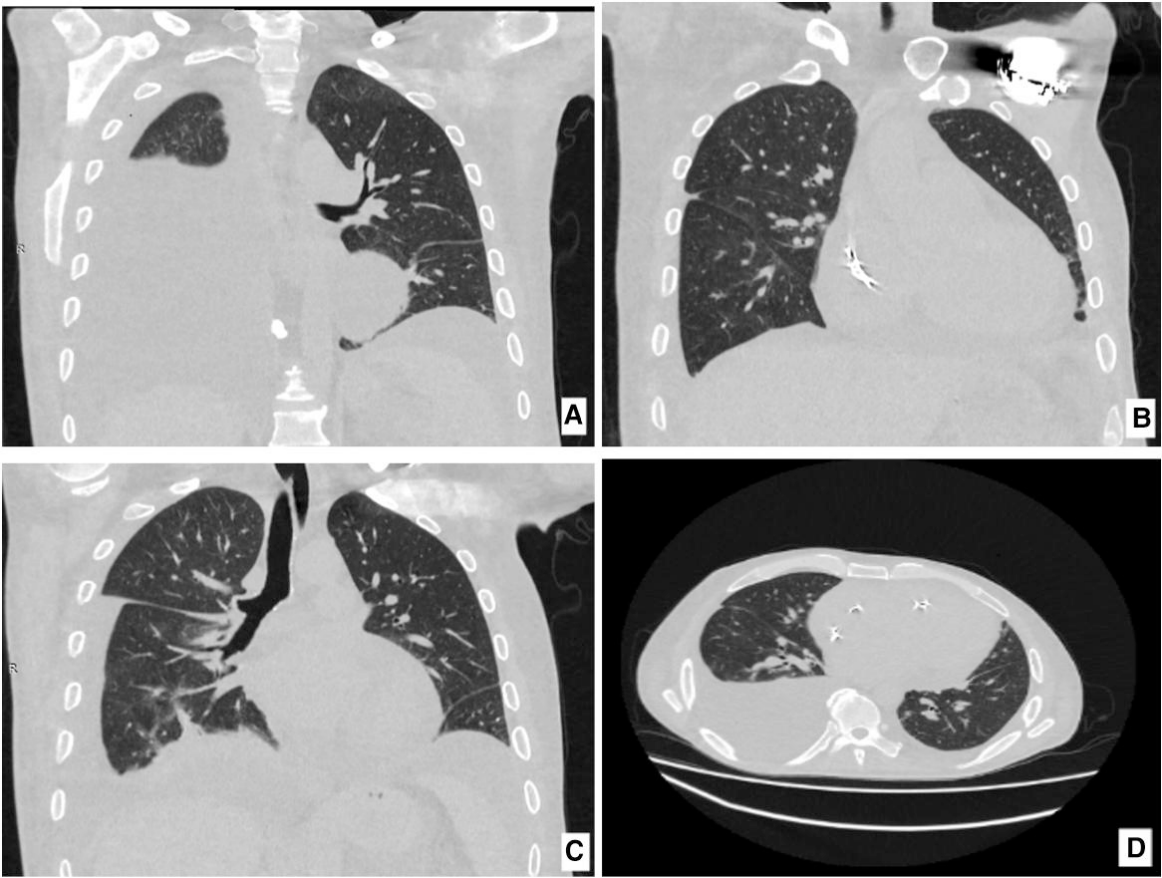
Pulmonary computed tomography. Pneumofibrotic changes and linear atelectasis in the bilateral basal pulmonary regions, free fluid in the bilateral pleural cavities (right: 1050 mL; left: minimal amount), free fluid in the pericardial cavity, and free fluid in the abdominal cavity.
